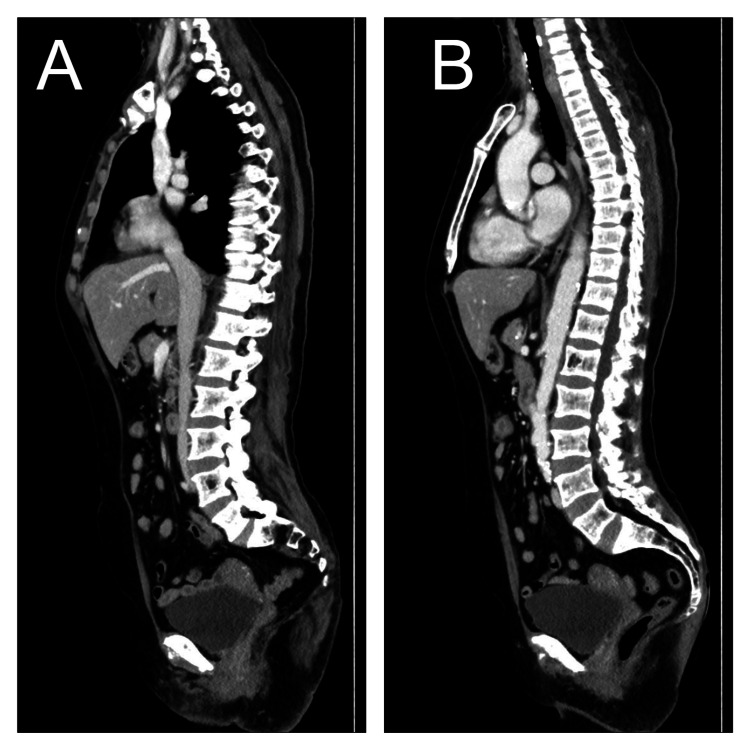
Abdominopelvic computed tomography imaging shows generalized marked osteopenia with small benign-looking osteolytic lesions involving lumbar vertebral bodies.
